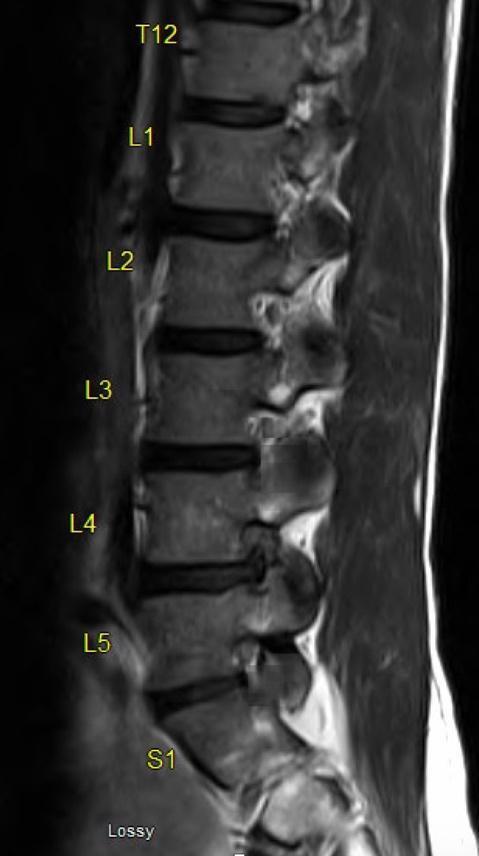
Figure 2 shows a T2 sagittal MRI of the lumbar spine with grade 0 or absence of stenosis at L2-3, grade 1 stenosis or mild foraminal stenosis at L3-4 with some fat obliteration in both the vertical and transverse directions, grade 2 stenosis or moderate foraminal stenosis at L5-S1 with perineural fat obliteration in all four directions without morphologic change in the nerve root, and grade 3 stenosis or severe foraminal stenosis at L4-5 showing complete perineural fat obliteration with nerve root collapse or deformation.
